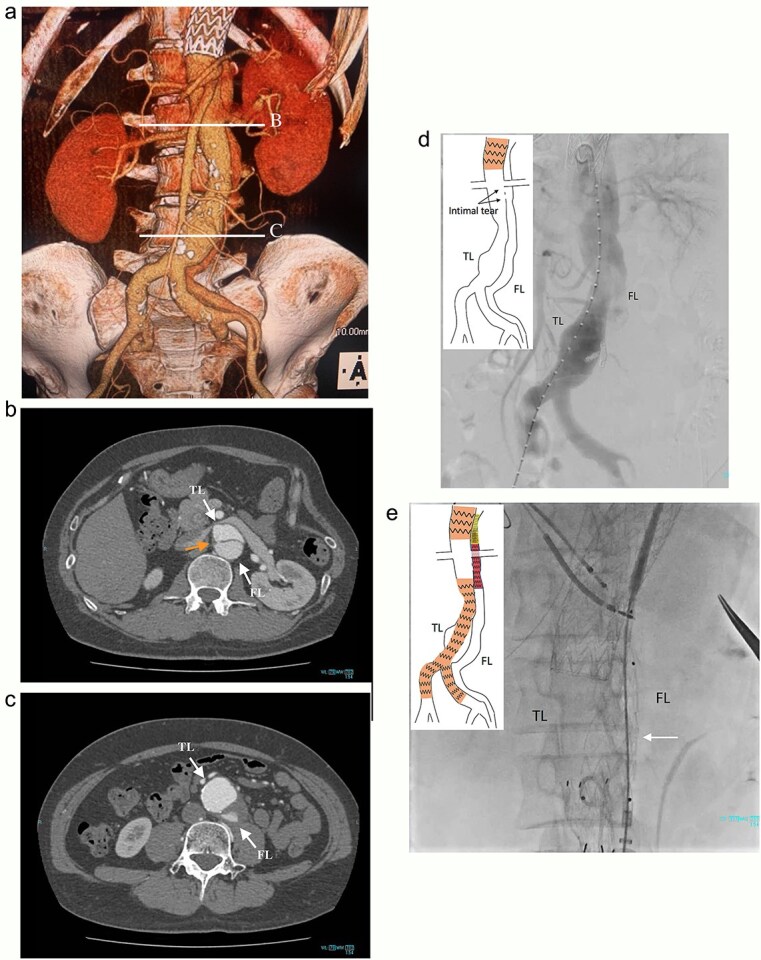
Preoperative CT 3D reconstruction showing the FL extended from the descending aorta to the external iliac artery (a), contrast-enhanced CT axial images at the renal artery level with an intimal tear (b; arrow), and the abdominal aortic aneurysm level (c), and the intraoperative angiography showing the FL were contrasted through intimal tear at the visceral segment (d), Endurant II iliac leg placed in the FL closing intimal tears near the renal artery (e; white arrow). TL: true lumen; FL: false lumen.
